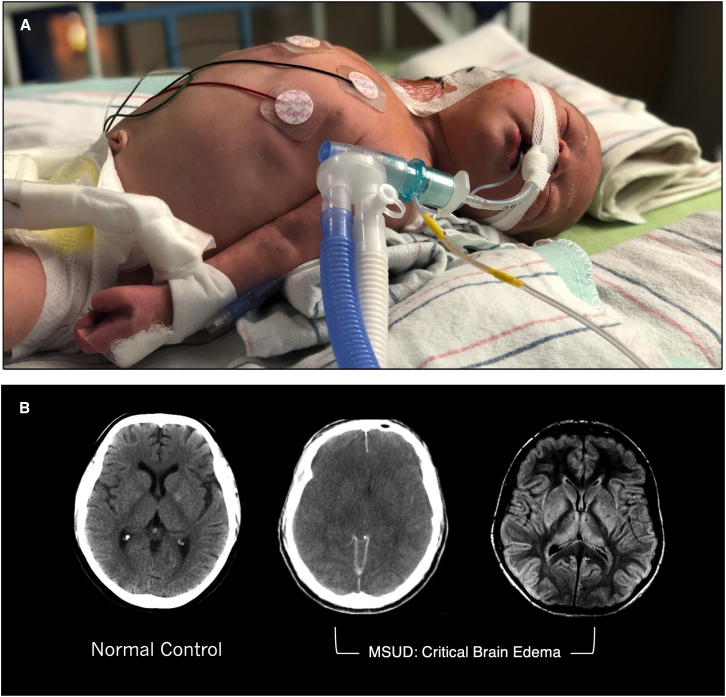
Perinatal metabolic intoxication (A) Opisthotonic posturing and central respiratory failure caused by neurochemical intoxication in MSUD; one of 11 children in the MATCH cohort experienced metabolic encephalopathy shortly after birth and was hospitalized for 18 days. (B) Metabolic intoxication is marked by severe brain swelling (computed tomography, middle) resulting from cytotoxic edema (MRI fluid-attenuated inversion recovery, right). For the one MATCH participant who died after a liver transplant, brain herniation from cerebral edema was the proximate cause of death. See also Table S5. Photo used with permission.
